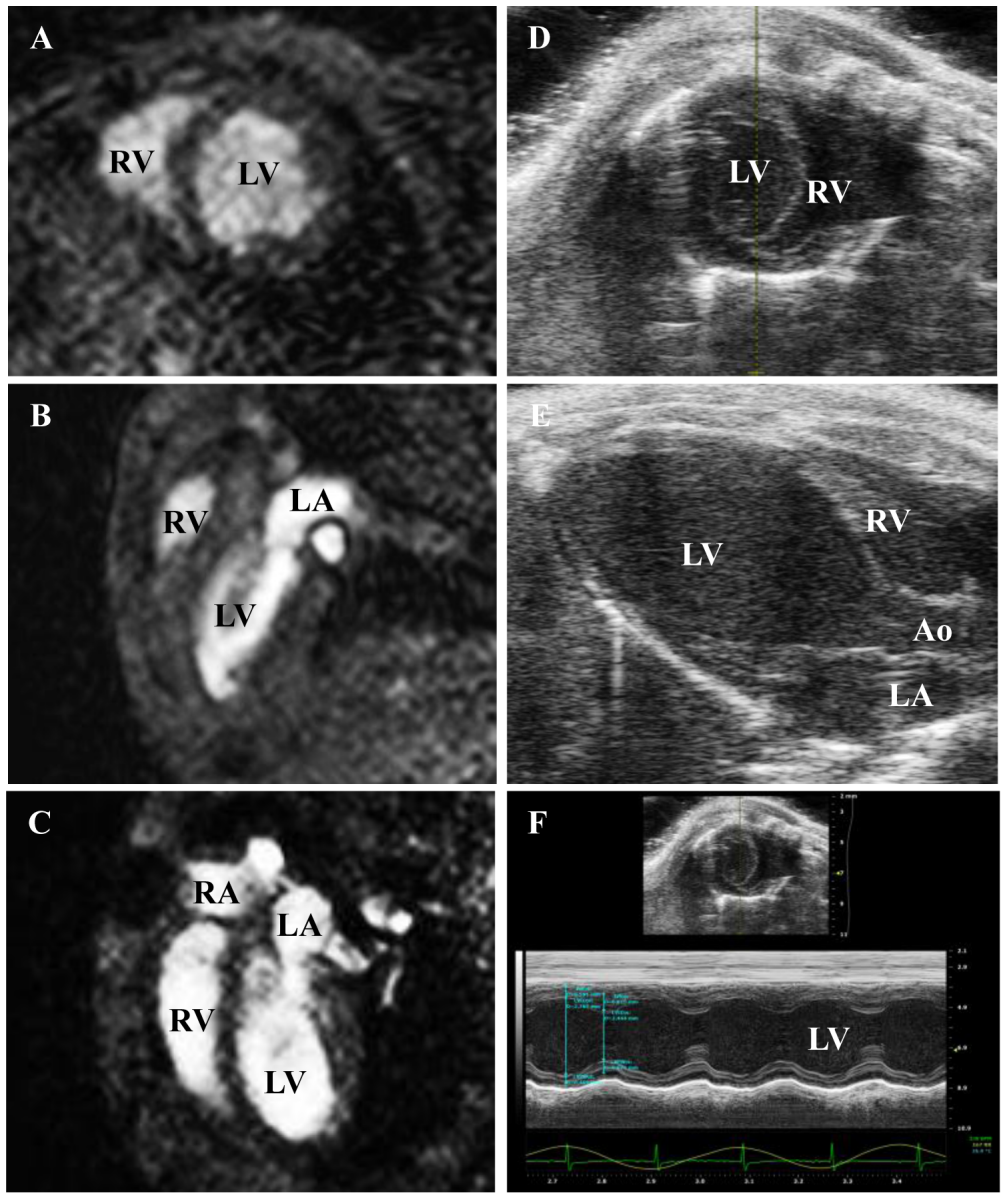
Representative slides of MRI and echocardiography-gained heart slides. Short axis view gained with A) MRI and D) echocardiography. Seven short axis slices were used for calculation of left ventricular volumes with MRI. B) Shows MRI-gained and E) echocardiography-gained long axis view. C) Shows 4-chamber view (MRI). F) Shows M-mode based calculation of LVEF (echocardiography). LV: left ventricle; RV: right ventricle; LA: left atrium; RA: right atrium; Ao: aorta.
